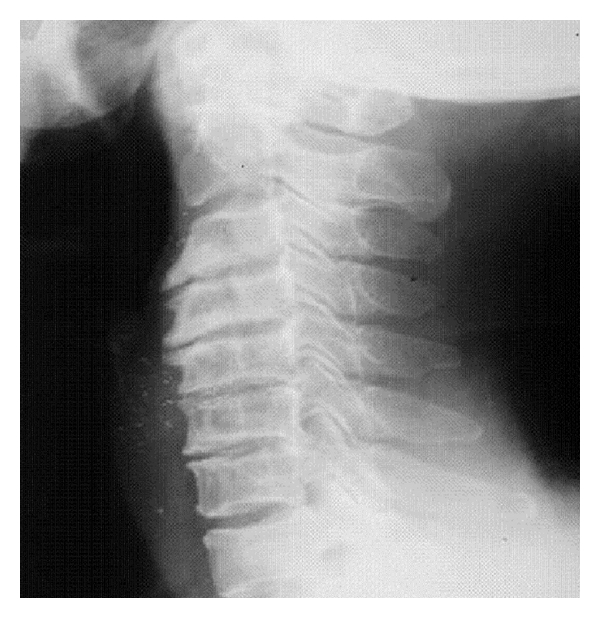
Radiographic representation of cervical spondylosis.
